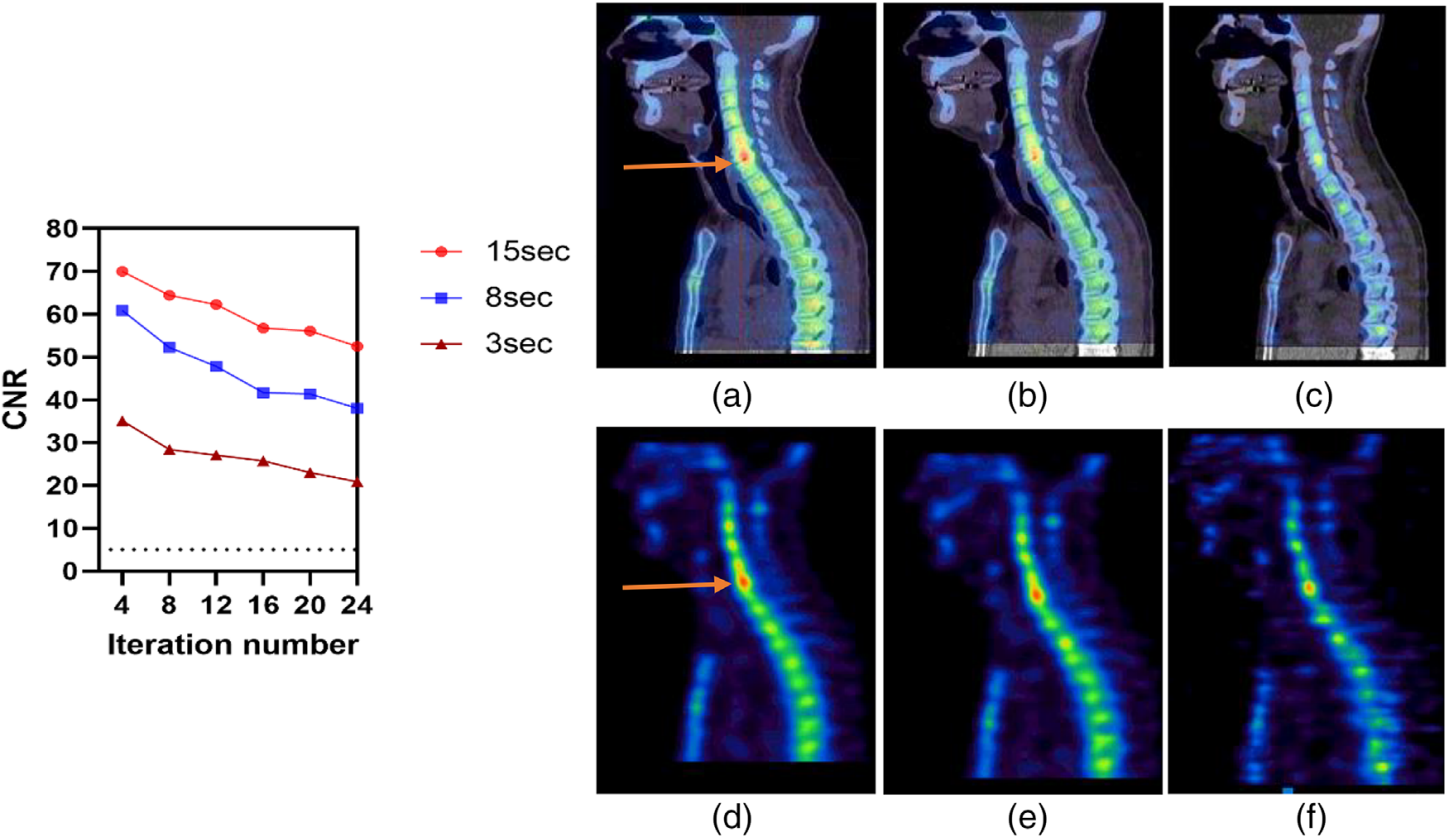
Left: contrast‐to‐noise ratio (CNR) for the abnormal uptake for the second clinical case at different acquisition time. Right: single‐photon emission computed tomography/computed tomography (SPECT/CT) and sagittal SPECT images at: (a and d) 15 s, (b and e) 8 s, and (c and f) 3 s. The dotted line on the graph represents CNR = 5 (Rose criteria). All reconstructions used 12 iterations, eight subsets, and an 8 mm Gaussian filter
